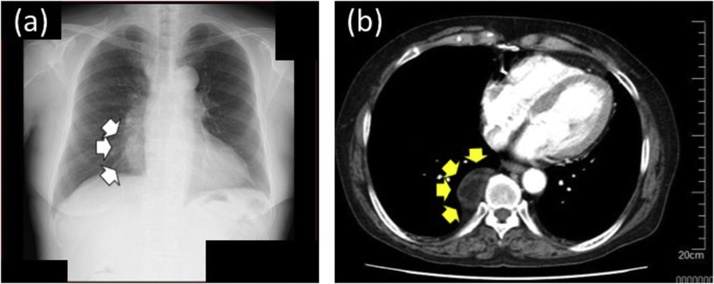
(A) Chest X-ray reveals an abnormal shadow in the right lower lung field (white arrows). (B) Chest CT reveals a 4.5-cm round tumor enhanced by contrast medium in the posterior mediastinal (yellow arrows).
